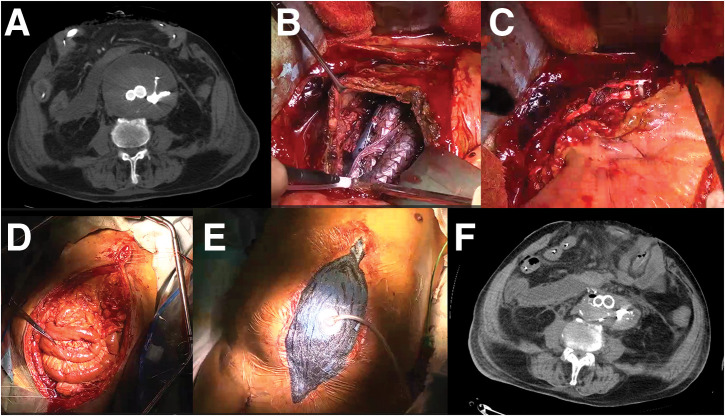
Open abdominal management after endovascular aortic repair. Reduced retroperitoneum hematoma after 2nd look procedure (A). Stent-graft inside an abdominal aortic aneurysm during aneurysmorrhaphy (B). Sutured aneurysm during aneurysmorrhaphy (C). Intraoperative findings showing difficulty in abdominal closure due to tissue edema (D). Intraoperative findings showing reapplication of negative pressure wound therapy for abdominal closure (E). Post-failed closure plain CT scan findings (F).
